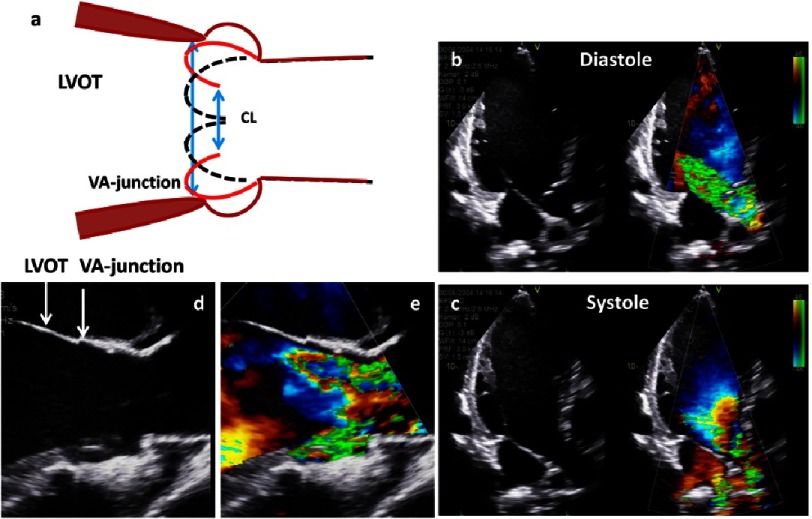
The scheme shows LV dilatation with dilatation of the LVOT and the basal aortic annulus and consecutive severe aortic regurgitation (a). Further, native and color-coded 2D transthoracic images during diastole (b) and systole (c) as well as native (d) and color-coded (e) 2D long axis views of the LVOT and the VA-junction during systole are shown.
